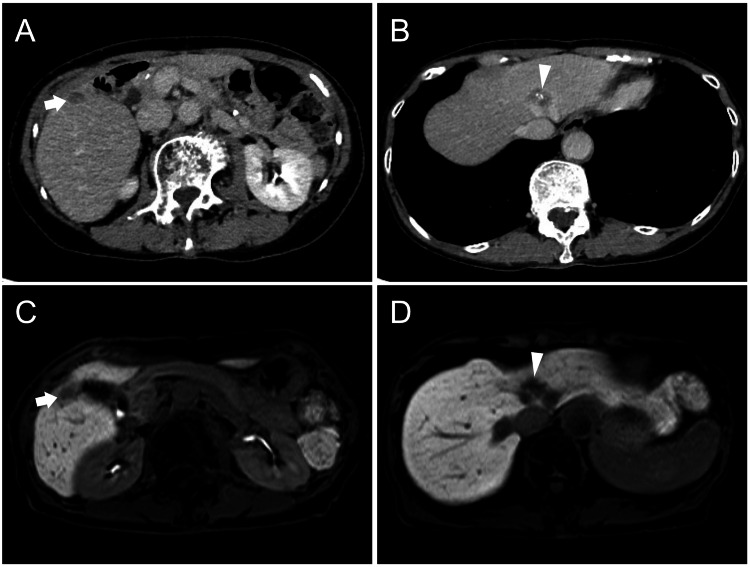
Diagnostic images before the second hepatectomy. Contrast-enhanced CT (A, B) and gadolinium ethoxybenzyl diethylenetriamine pentaacetic acid-enhanced MRI (C, D): The lesion in segment V (arrow) is oval-shaped and slightly enlarged (A); however, the lesion in segment IV (arrowhead) has markedly shrunk in response to chemotherapy (B). The lesion in segment V appears to be within the liver parenchyma (C). The lesion in segment IV is in contact with the root of the middle hepatic vein and the left hepatic vein (D).
